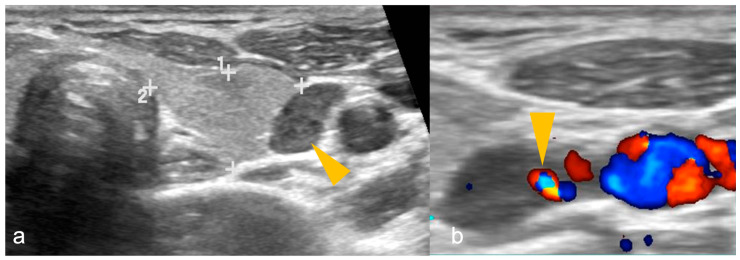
(a,b): Parathyroid adenoma on ultrasonography in a patient with primary hyperparathyroidism. (a) A well-defined oval-shaped homogeneously hypoechoic lesion (arrowhead) lateral to the left lobe of thyroid gland (shown by 1, 2 and + sign). (b) Colour Doppler image shows feeding vessel sign (arrowhead). Imaging findings are suggestive of parathyroid adenoma.
